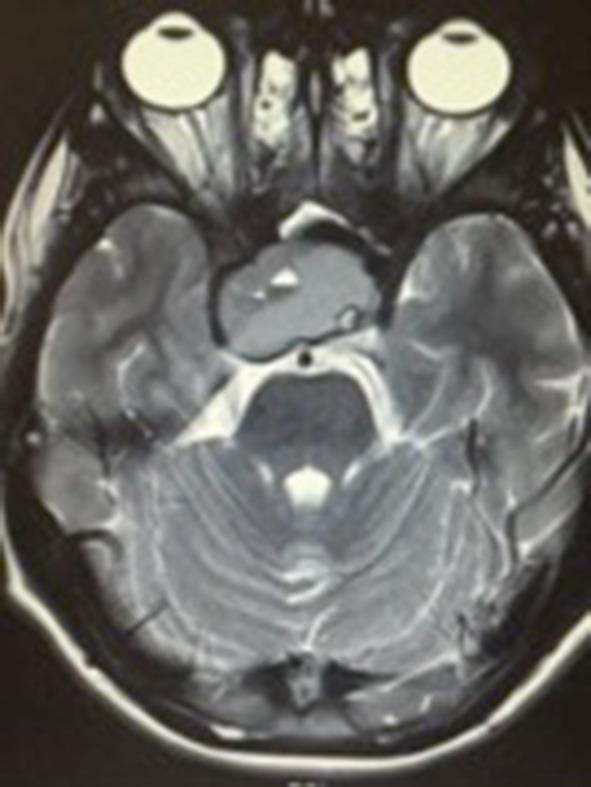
Child 5’s T2 weighted MRI showing a large suprasellar mass with right parasellar extension, displacing the right internal carotid artery. There is evidence of central necrotic change and blood-fluid levels
